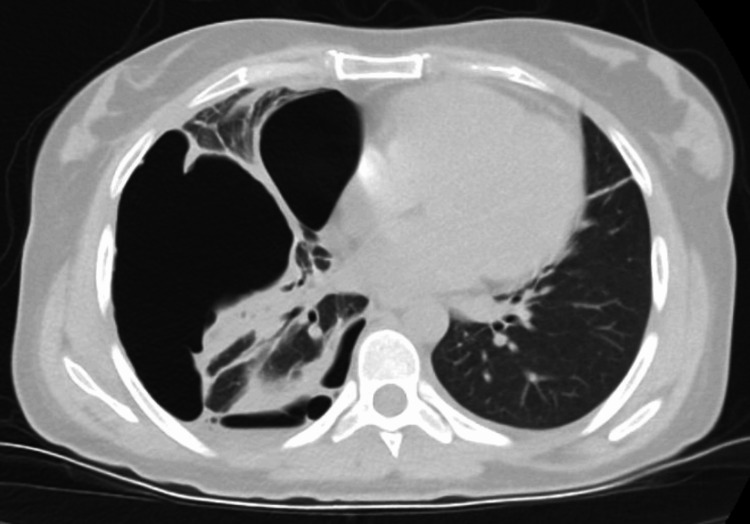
Pulmonary CT angiography showing localized pleurisy on the right, fluid in the right interlobar fissure, and parenchymal collapse in the lower zones of both lungs.
